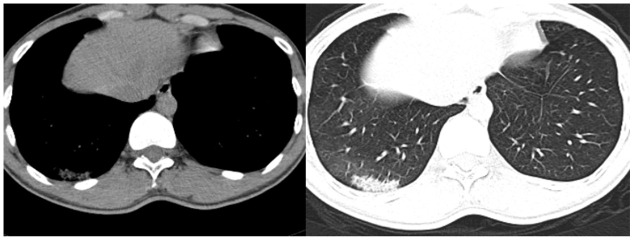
Chest CT examination of the patient on postoperative day 3.
